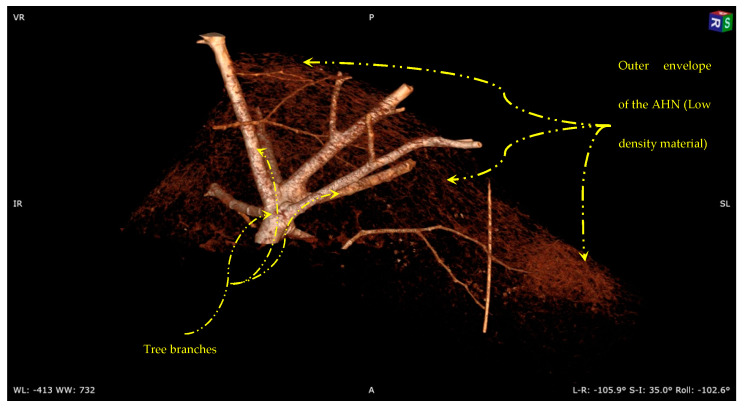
CT scan image shows the outer envelope of the Asian hornet nest (tree branches and paper-like material with low density), the patient orientation in space: (S)—superior and (R)—right.
